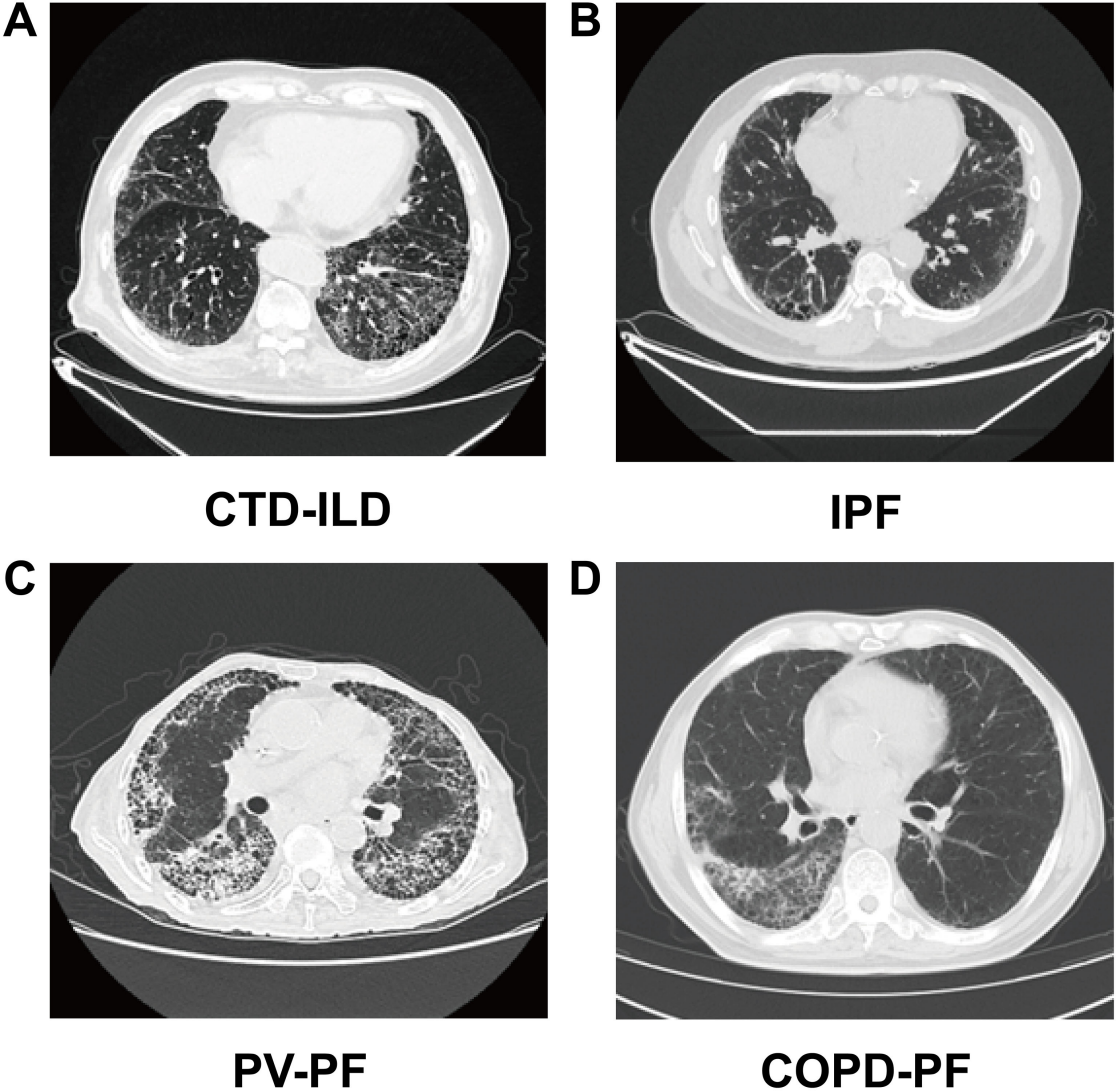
Representative high-resolution computed tomography (HRCT) images of fibrotic interstitial lung diseases (ILDs) with distinct etiologies. (A) connective tissue disease–associated interstitial lung disease (CTD-ILD): Nonspecific interstitial pneumonia (NSIP) pattern characterized by ground-glass opacities (GGO), reticulation, and traction bronchiectasis with symmetric involvement of the lower lobes. (B) idiopathic pulmonary fibrosis (IPF): Usual interstitial pneumonia (UIP) pattern showing subpleural honeycombing, traction bronchiectasis, and basal predominance. (C) post-viral pulmonary fibrosis (PV-PF): Pulmonary vasculopathy-associated pulmonary fibrosis with early-stage ground-glass opacities and crazy paving, progressing to reticulation, volume loss, and atypical fibrotic changes. (D) chronic obstructive pulmonary disease–associated pulmonary fibrosis (COPD-PF): Combined pulmonary fibrosis and emphysema with upper lobe–predominant emphysema and patchy, asymmetric fibrosis in the lower lobes.
